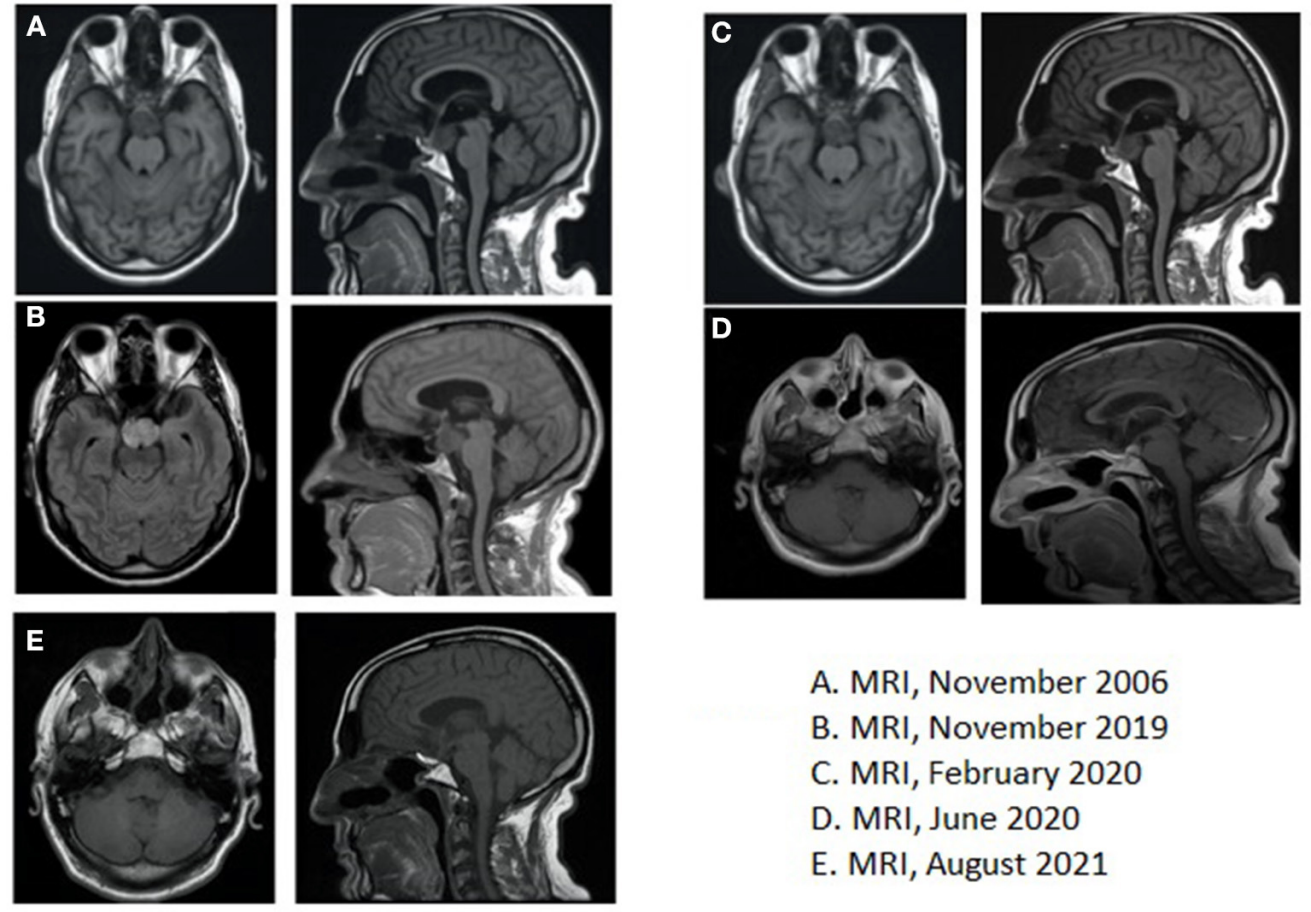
(A) Brain magnetic resonance imaging (MRI), November 2006, at the time of the first diagnosis; (B) Brain MRI, November 2019, at the time of recurrence; (C) Brain MRI, February 2020, showing residual disease after post-second surgery, before starting medical therapy with BRAF- and MEK-inhibitors; (D) Brain MRI, June 2020, at the time of partial response after 4 months of medical therapy; (E) Brain MRI, August 2021, after 18 months of medical therapy.
